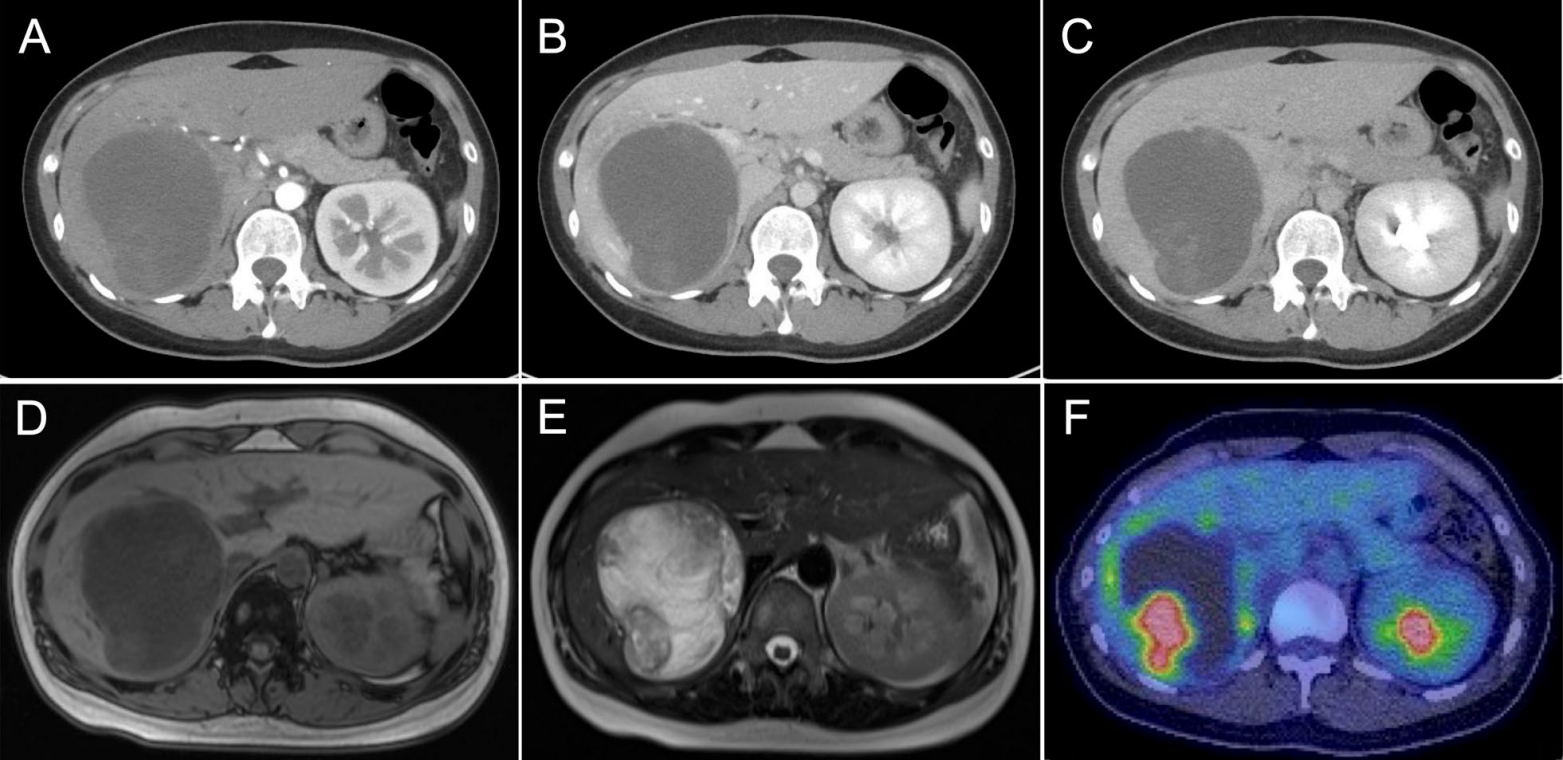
Preoperative imaging findings. CT imaging demonstrating the hepatic tumor (axial section): A arterial phase, B portal phase, and C equilibrium phase. MRI imaging demonstrating the hepatic tumor: D T1-weighted and E T2-weighted images. F Positron emission tomography (PET) imaging
